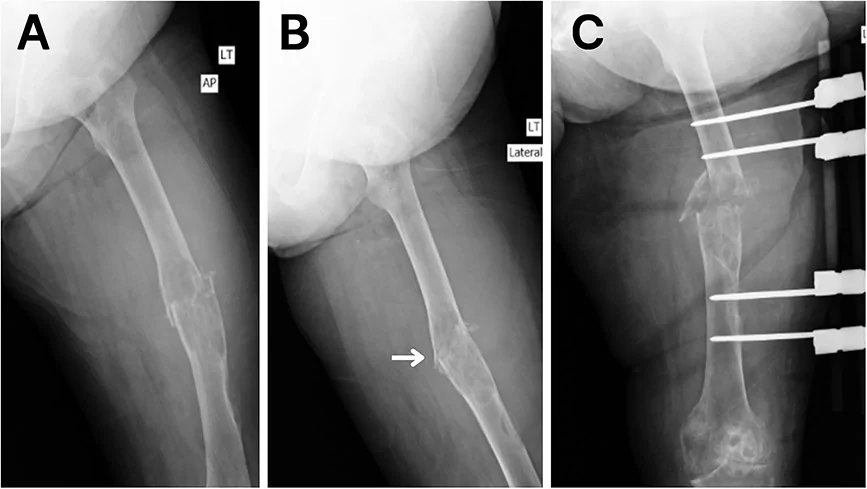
(A) AP view X-ray of the left femur. (B) AP view X-ray of the left femur, which shows a displaced fracture in the shaft of the femur (arrow). (C) AP view X-ray of the left femur shows the plates and screws used to fix the fracture.
